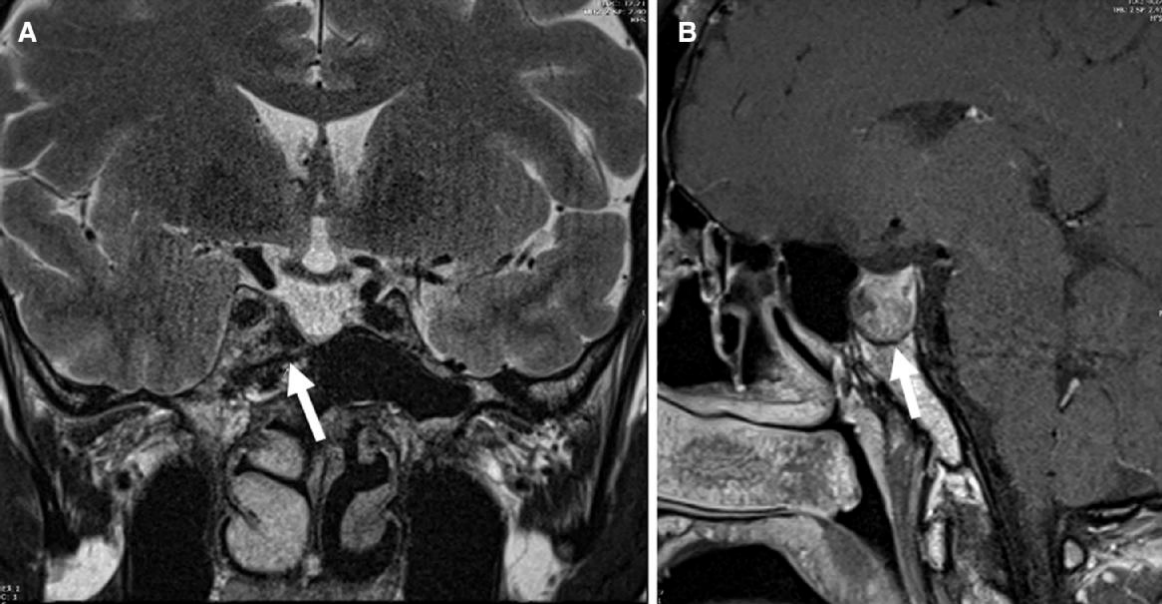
Cross-sectional (A) and sagittal (B) MRI scans showing residual pituitary tumor (December 2021).
